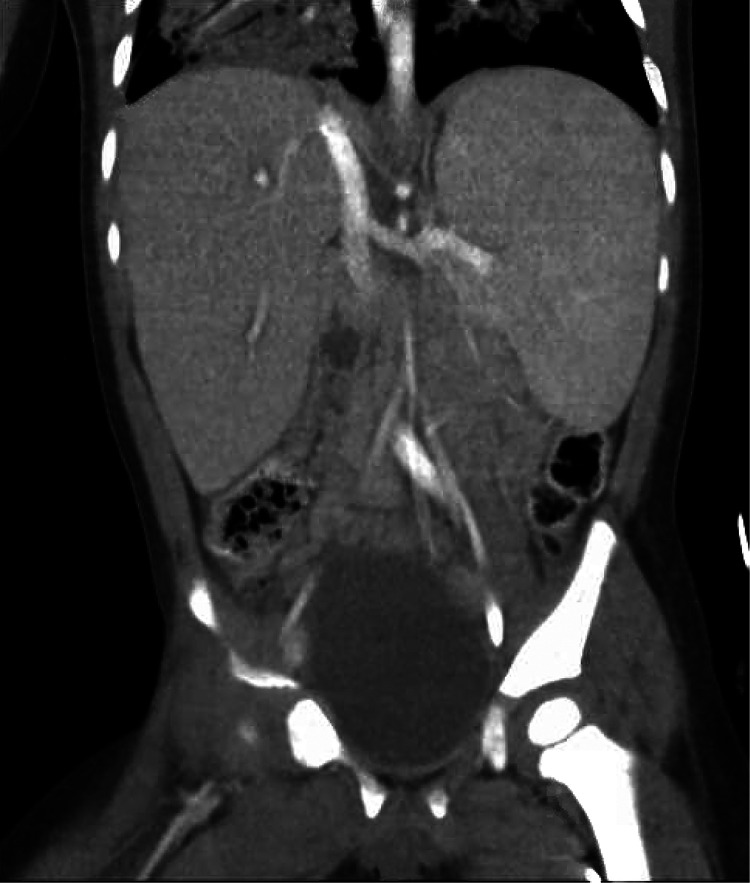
Abdominal computed tomography scan done on hospital day 2 demonstrating marked hepatosplenomegaly.
